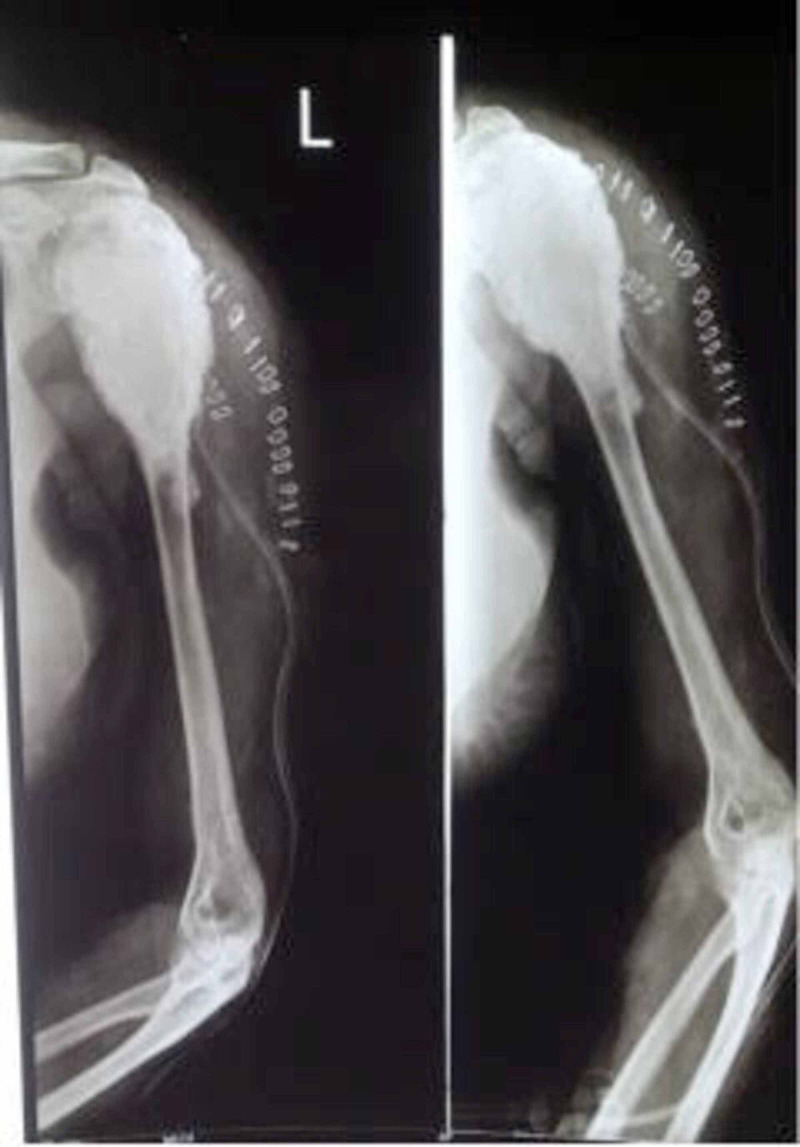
Postoperative X-ray showing the second recurrence managed by curettage and antibiotic cementation (2008)
